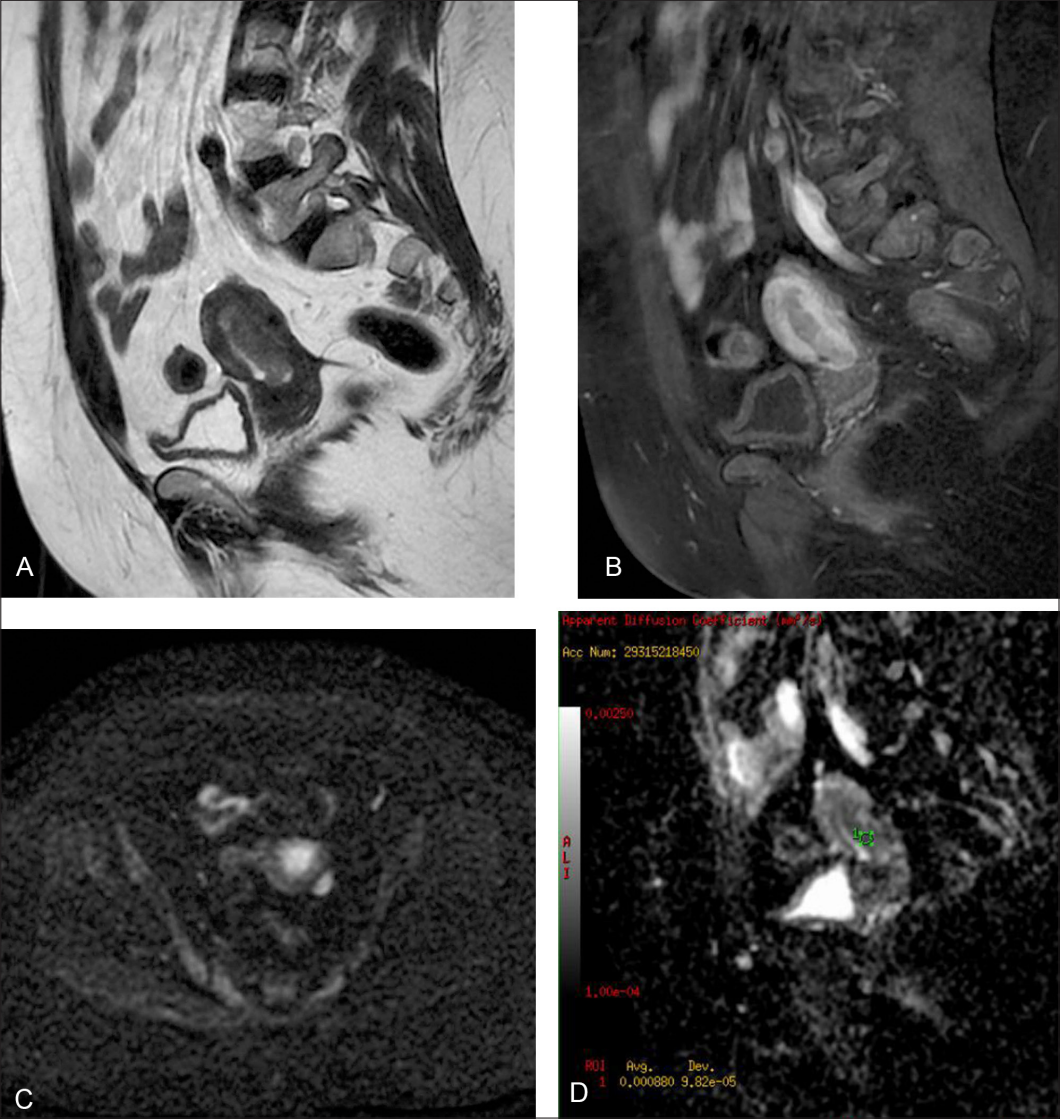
A 56-year-old woman with endometrial cancer. Sagittal T2-weighted fast spin-echo image showing a hyperintense mass in the endometrial cavity (a). Sagittal gadolinium-enhanced fat-saturated spin-echo T1-weighted image shows the tumor as a slightly enhanced lesion (b). Axial diffusion weighted image (b = 1000s/mm^2^) (c) shows hyperintense FIGO stage 1A endometrial mass. The ADC value is 0.88x10^–3^ on the sagittal ADC map image (d).
